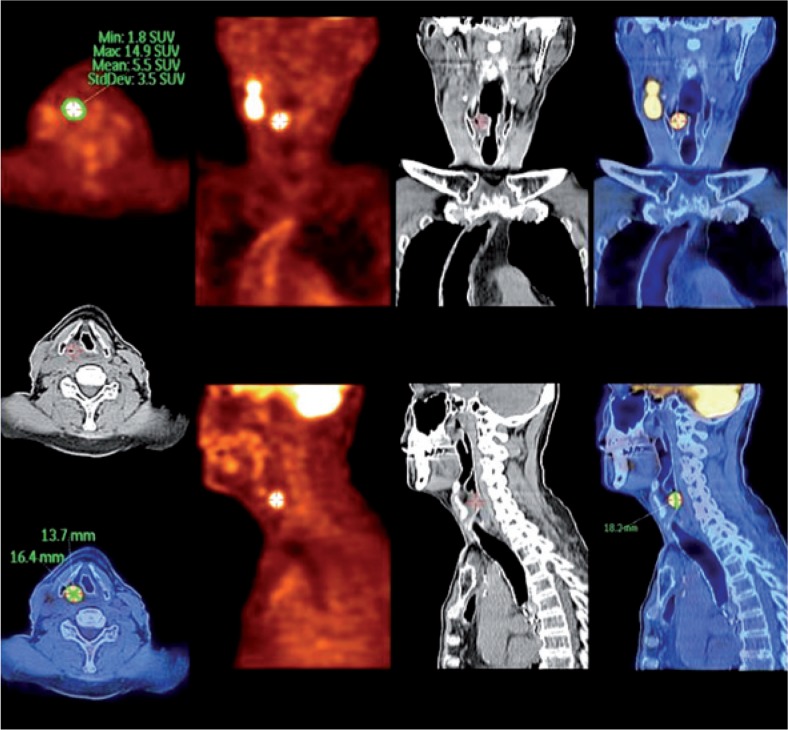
PET-CT images of 51-year old male with metastatic squamous cell carcinoma of unknown origin in cervical lymph nodes. The origin was found by PET-CT and later confirmed by a biopsy.
